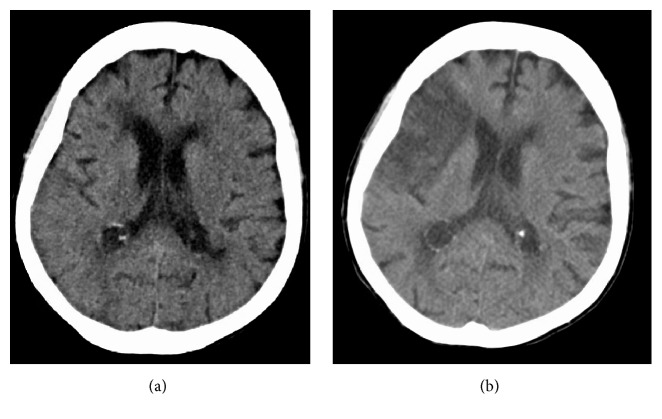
CT scan of the head performed on admission (a) compared to one performed after 14 days in the ICU (b) shows development of a large right MCA infarct.
